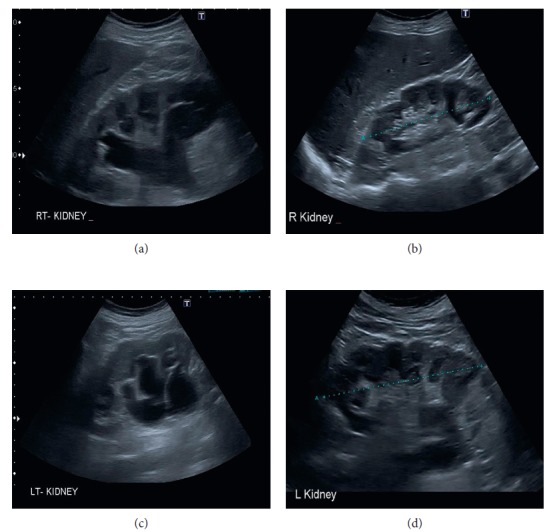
Preoperative ultrasound scan of the right kidney showing hydronephrosis (a) and resolution postoperatively (b). Hydronephrosis of left kidney (c) and postoperative resolution (d). Cortical cysts are seen.
